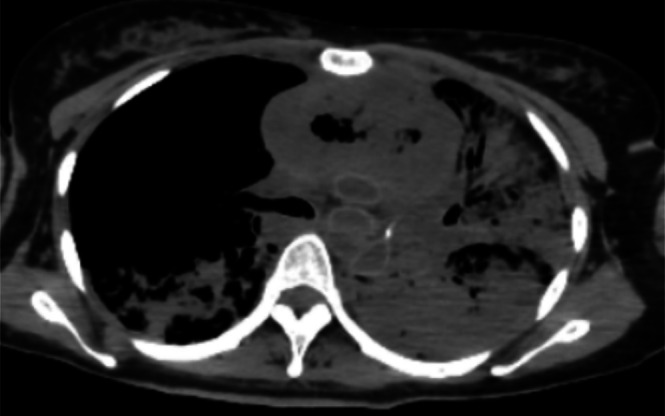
Post‐mortem chest computed tomography. A large amount of air was observed within the mediastinal tumor. Blood had accumulated in the trachea, and the left bronchus was completely obstructed by blood. Findings consistent with aspiration pneumonia were noted, mainly in the left lung field, which showed almost no aeration.
